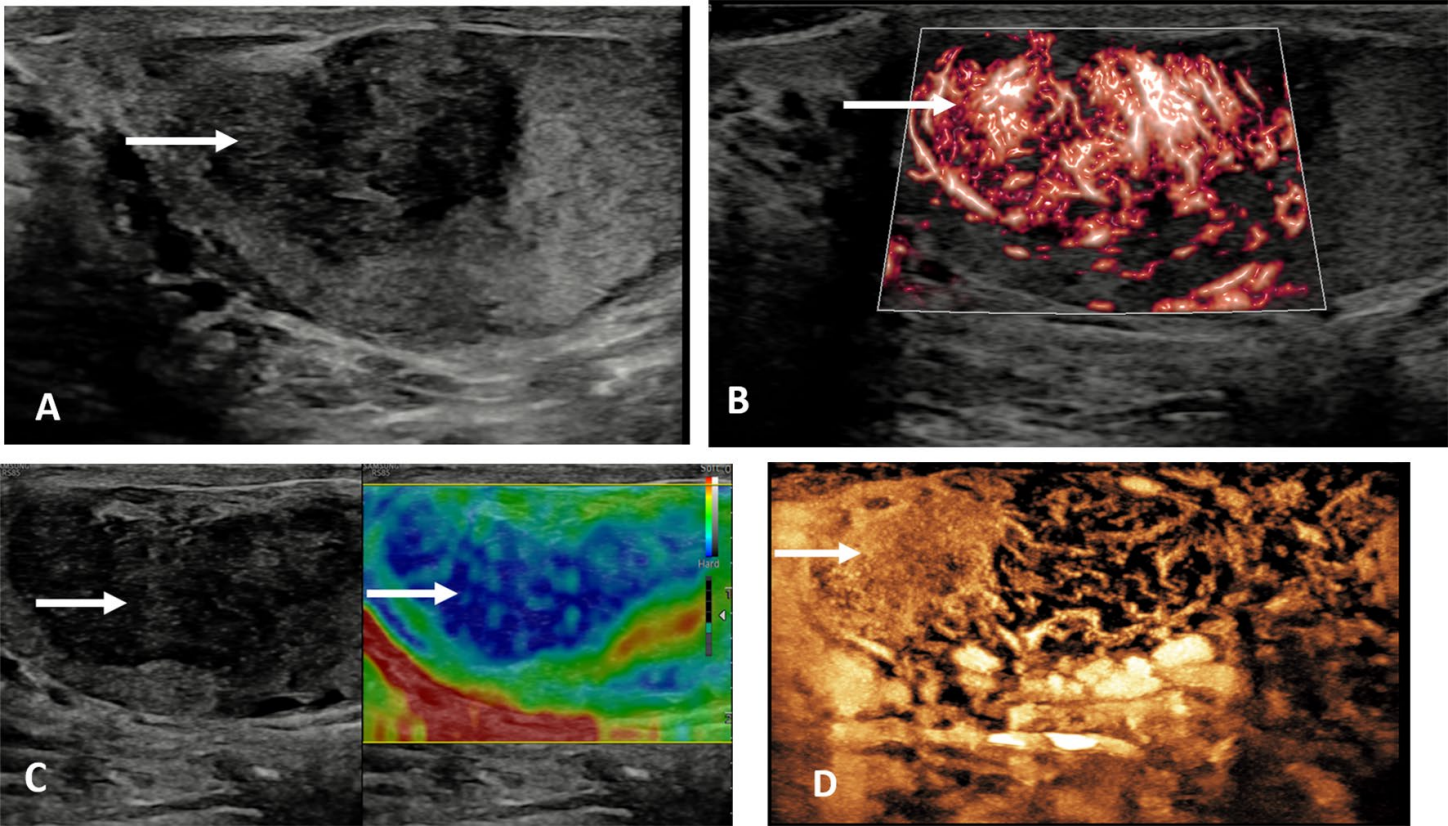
A 18-year old with known congenital adrenal hyperplasia undergoing screening ultrasound of the testis with bilateral adrenal rest cells noted. A Longitudinal view of the right testis on B-mode demonstrating a focal low reflective intra-testicular tumor (arrow) mimicking a primary germ cell tumor. B The microvascular image demonstrating color flow (arrow) within the tumor, disordered and intense in comparison to the normal testis parenchyma. C The tissue elastography image shows that the lesion is stiffer than the surrounding normal tissue (arrows). D A contrast enhanced ultrasound examination, with maximum intensity projection, demonstrates a highly vascular lesion (arrow)
